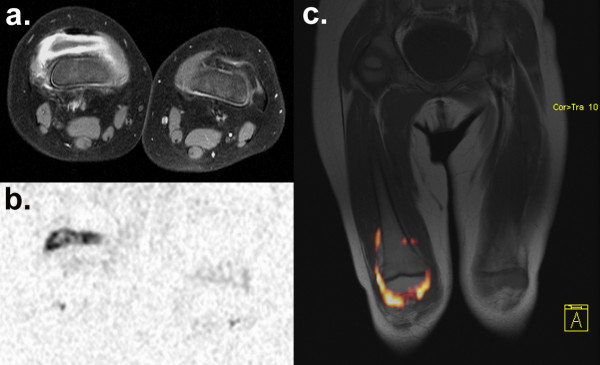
Diffusion-weighted imaging of synovitis. Gonarthritis of the right knee in a 2-year-old girl with juvenile idiopathic arthritis (JIA). Marked synovial thickening and contrast enhancement accompanied by joint effusion are seen on transversal T1W TSE FS (a). DWI at b = 1000 with inverted gray scale depicts synovitis based on restricted diffusion on a corresponding transversal section acquired with 2 averages and 41s scan time (b). Joint effusion shows low signal on DWI and can be distinguished from the surrounding layer of synovitis. The unaffected left knee does not show inflammatory signal alterations on contrast-enhanced T1W imaging, nor on DWI. Overlay of colourised DWI b = 1000 data on coronal pre-contrast T1W TSE (c) with semi-automatic 3D image fusion.
